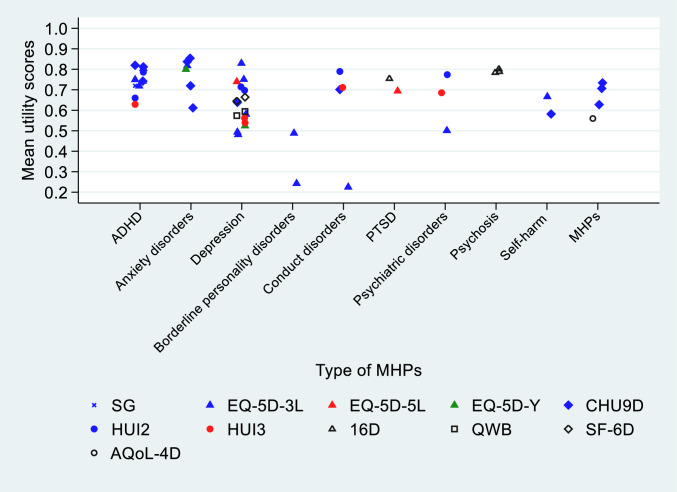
Reported HSUVs by type of elicitation tools across MHPs. Notes: MHPs having HSUVs derived from only one study were excluded (internalising problems, medicine use, delinquency, tobacco use, other drug used disorders, avoidant personality disorder, personality disorder, depressive personality disorder, and obsessive-compulsive personality disorder)
